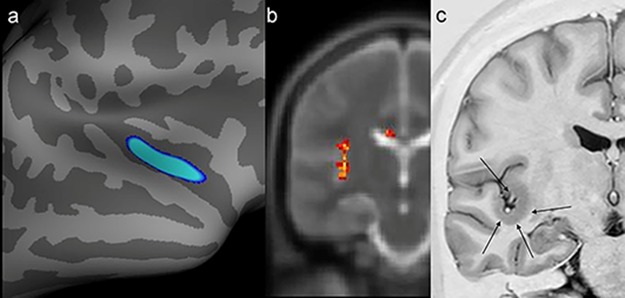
Example of a patient who switched to positive magnetic resonance imaging (MRI) status only after targeted visual evaluation guided by postprocessing (patient 19). A possible epileptogenic zone had not been regionalized in this patient with video electroencephalography. However, he exhibited abnormalities in the same region by two quantitative measures. a) Postprocessing of cortical thickness (quadrature decoder [QDEC]) showed temporo-insular thickening in the right hemisphere (blue image). b) Evaluation of T2 relaxometry maps with Statistic Parametric Mapping (SPM12) software also revealed a signal abnormality in the same location (yellow and red foci). c) Coronal T2 short tau inversion recovery (STIR) (inverted window) showing cortical thickening in the same region (black arrows).
